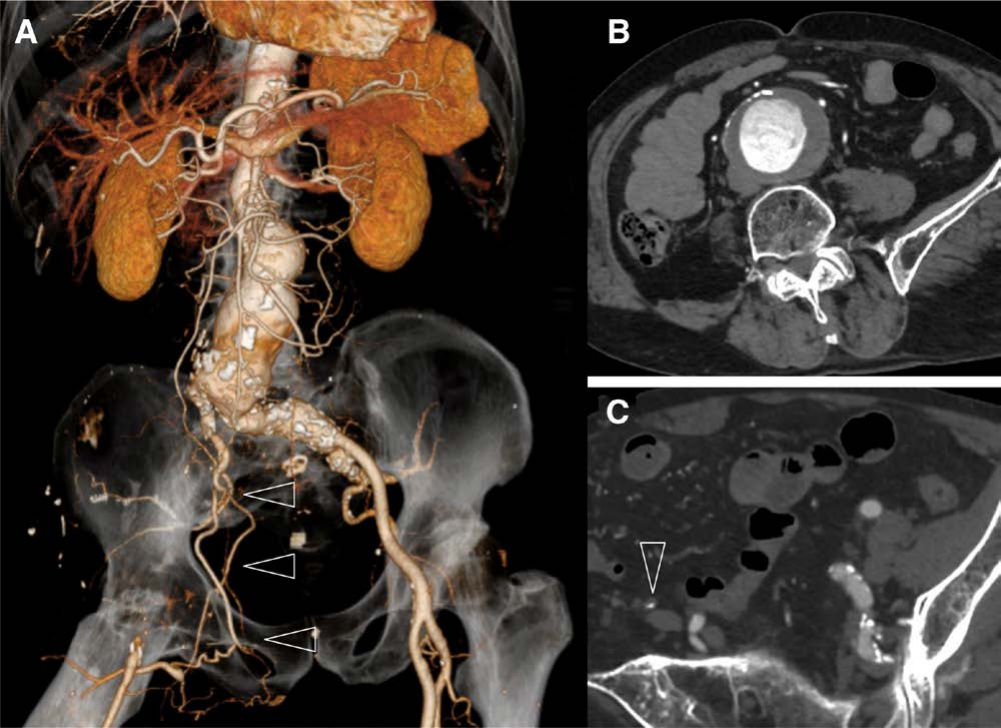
Preoperative enhanced CT images. (A) Volume rendering image of CT angiography showing occlusion of the right EIA to the CFA with collateral flow from the right IIA (arrow heads). (B) Axial view of CT shows AAA. (C) Contrast enhanced CT showing occlusion of the right EIA. The EIA was atrophied (arrowhead). AAA = abdominal aortic aneurysms, CFA = common femoral artery, CT = computed tomography, EIA = external iliac artery.
